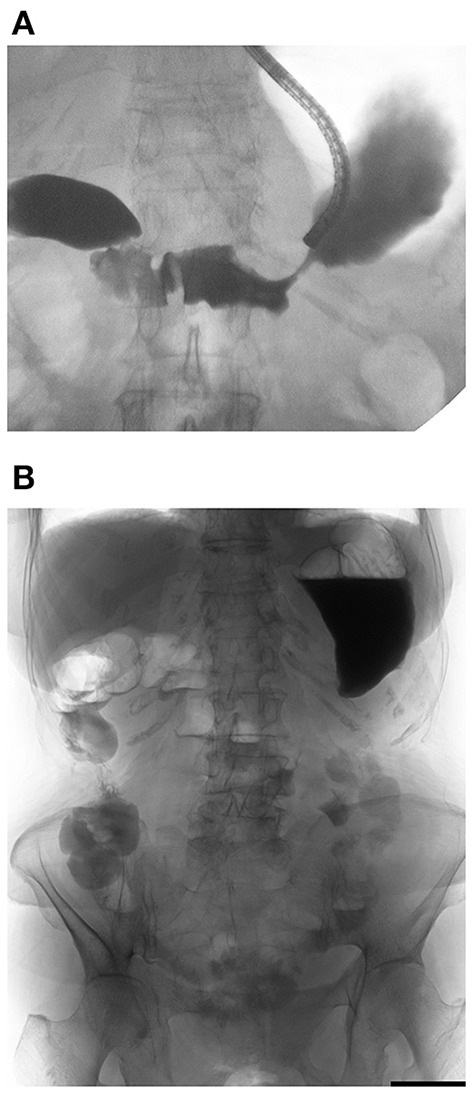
Water-soluble contrast challenge. (A) Water-soluble contrast was injected beyond the obstruction. (B) Plain X-ray taken 4 h after injection showed contrast reached the large intestine, which means well small bowel transit without the coexistence of small-bowel obstruction.
